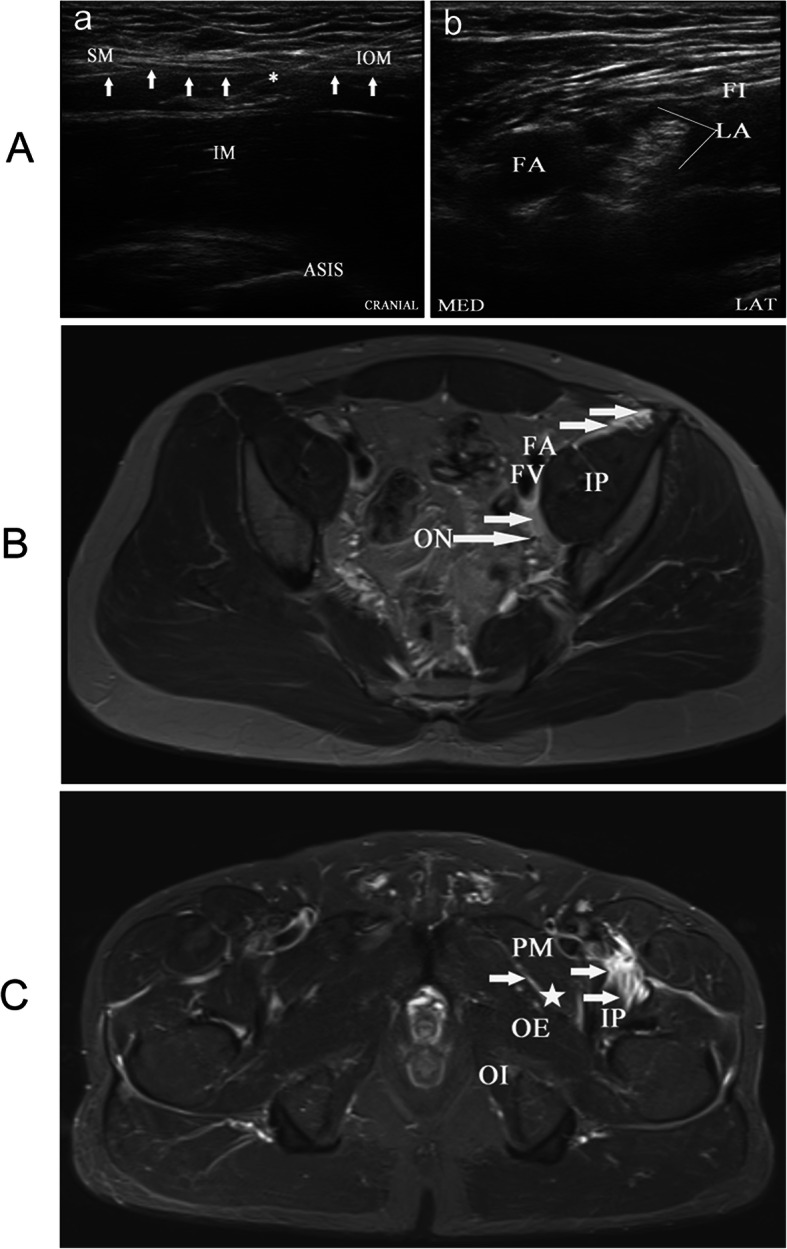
Ultrasound and magnetic resonance images. a. Ultrasound images of a novel suprainguinal fascia iliaca compartment block (FICB). (a) Ultrasound image for identification of the relevant structures for FICB. White arrows, fascia iliaca; *, needle; ASIS, anterior superior iliac spine; SM, sartorius muscle; IOM, internal oblique muscle; IM, iliac muscle; (b) local anesthetic around the FN. FI, fascia iliaca; FN, femoral nerve; FA, femoral artery; MED, medial; LAT, lateral. b. An axial T2- weighted fat-suppressed magnetic resonance image at the level of the fourth sacral vertebra shows medial spread of injectate (small white arrows) in a plane superficial to the IP muscle and deep to the FA and FV. IP: iliopsoas; ON: obturator nerve; FA: femoral artery; FV: femoral vein. c. An axial T2-weighted fatsuppressed magnetic resonance image at the level of the second coccygeal vertebra shows medial spread of injectate (white arrows) in a plane superficial to the IP muscle and diffuses below the pectineus muscle to obturator nerve (☆). PM: pectineus muscle; IP: iliopsoas; OE: obturator externus; OI: obturator internus
